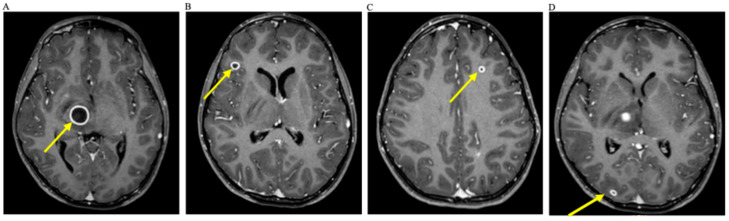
Brain localization of multiple abscesses on MRI at thalamus (A), right frontal lobe (B), left frontal lobe (C), and right occipital lobe (D), respectively (see arrows).
